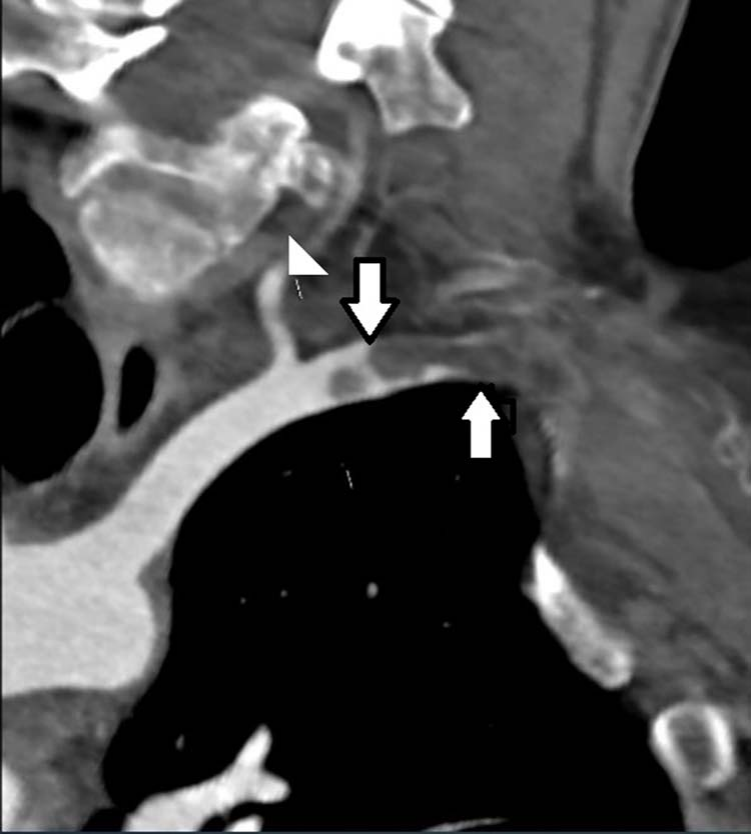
CT angiogram demonstrating location of the thoracic outlet obstruction (up arrow) and retrograde thrombosis (down arrow).
